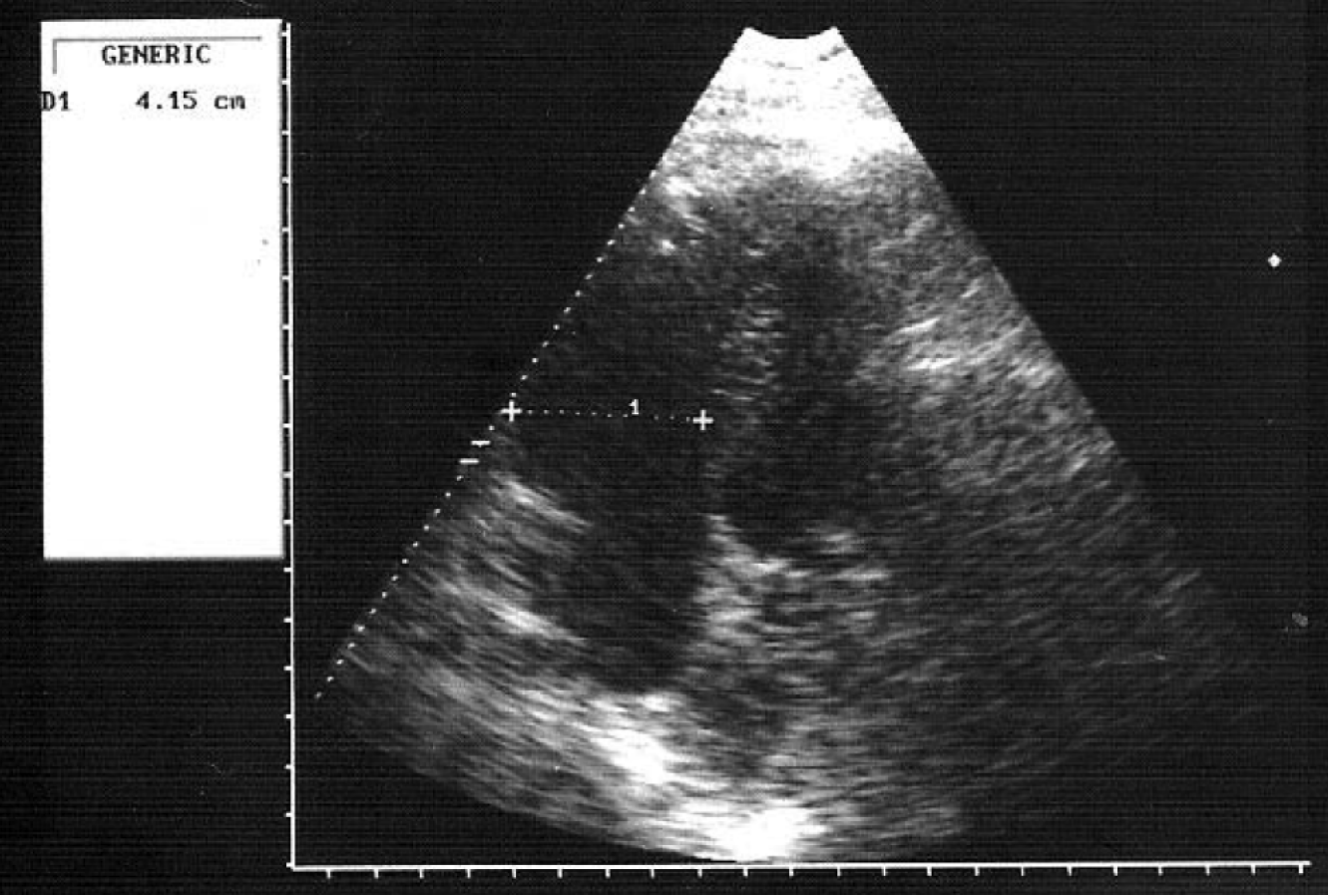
Apical 4-chamber view revealing a markedly dilated right ventricle with an end-diastolic diameter of 4.15 cm. There is no evidence of right ventricular or left ventricular thrombus.
